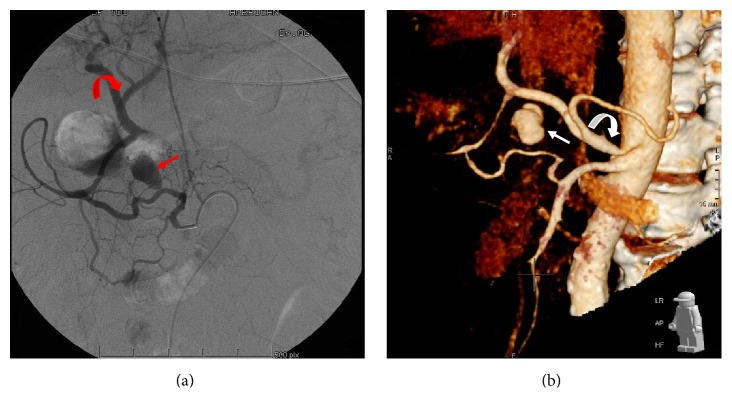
Selective digital subtraction angiography (DSA) of the superior mesenteric artery*: (a) A 2.0- × 1.4-cm narrow neck oblong aneurysm* (Arrow)* originating from the anterior branch of the inferior pancreaticoduodenal artery. Note the several collaterals originating from the superior mesenteric artery and the retrograde filling of the hepatic artery* (Curved arrow)* through the pancreaticoduodenal arcade. (b) Three-dimensional reconstruction of the enhanced CT images revealing the oblong aneurysm* (Arrow)*, originating from a branch of the superior mesenteric artery. Note the stenosis at the origin of the celiac trunk* (Curved arrow).*
